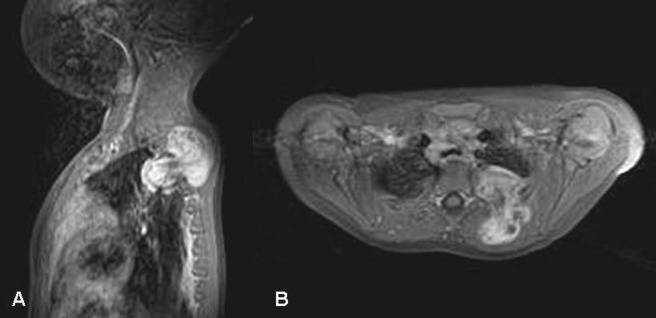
Figure 1: T1-weighted sagittal (A) and axial (B) MRI of the cervico-dorsal region showing a hyperintense well-circumscribed mass arising from suprascapular soft tissue and growing through the ribs into the left chest cavity.
